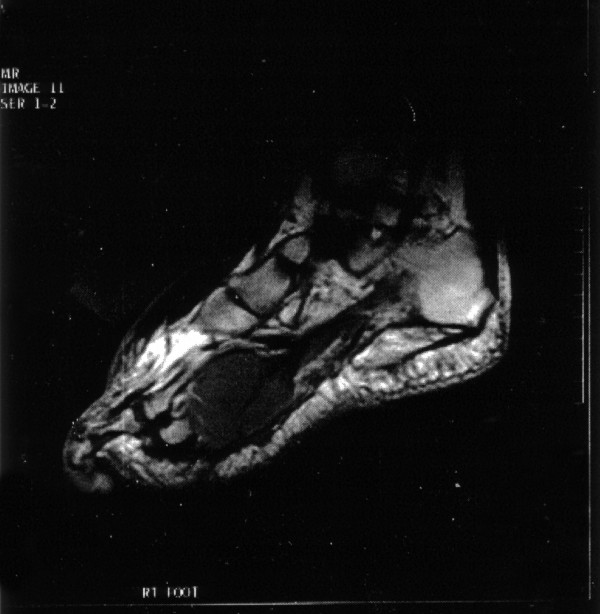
MRI of the right foot in Case 2. A 7 × 5 cm mass is present involving the skin and flexor tendons.
